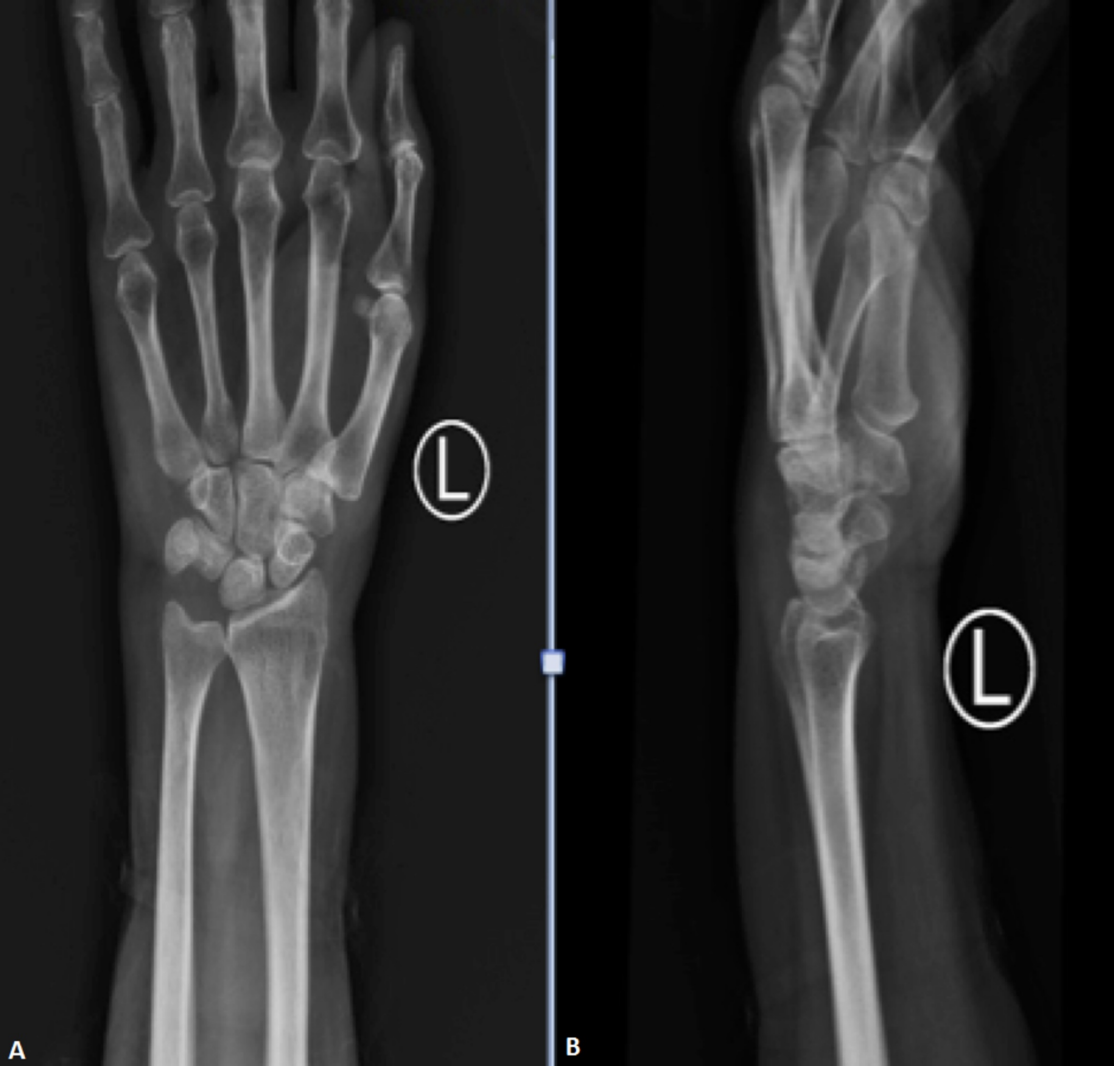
X-ray film of the left wrist on admission; increased soft tissue shadow with no obvious abnormality A: antero-posterior view; B: lateral view
